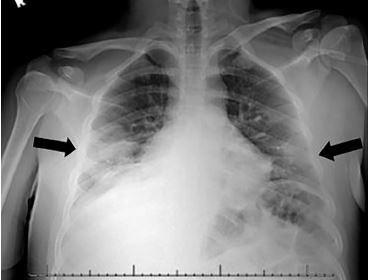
A chest radiograph demonstrating bilateral infiltrates consistent with bilateral multifocal pneumonia.
